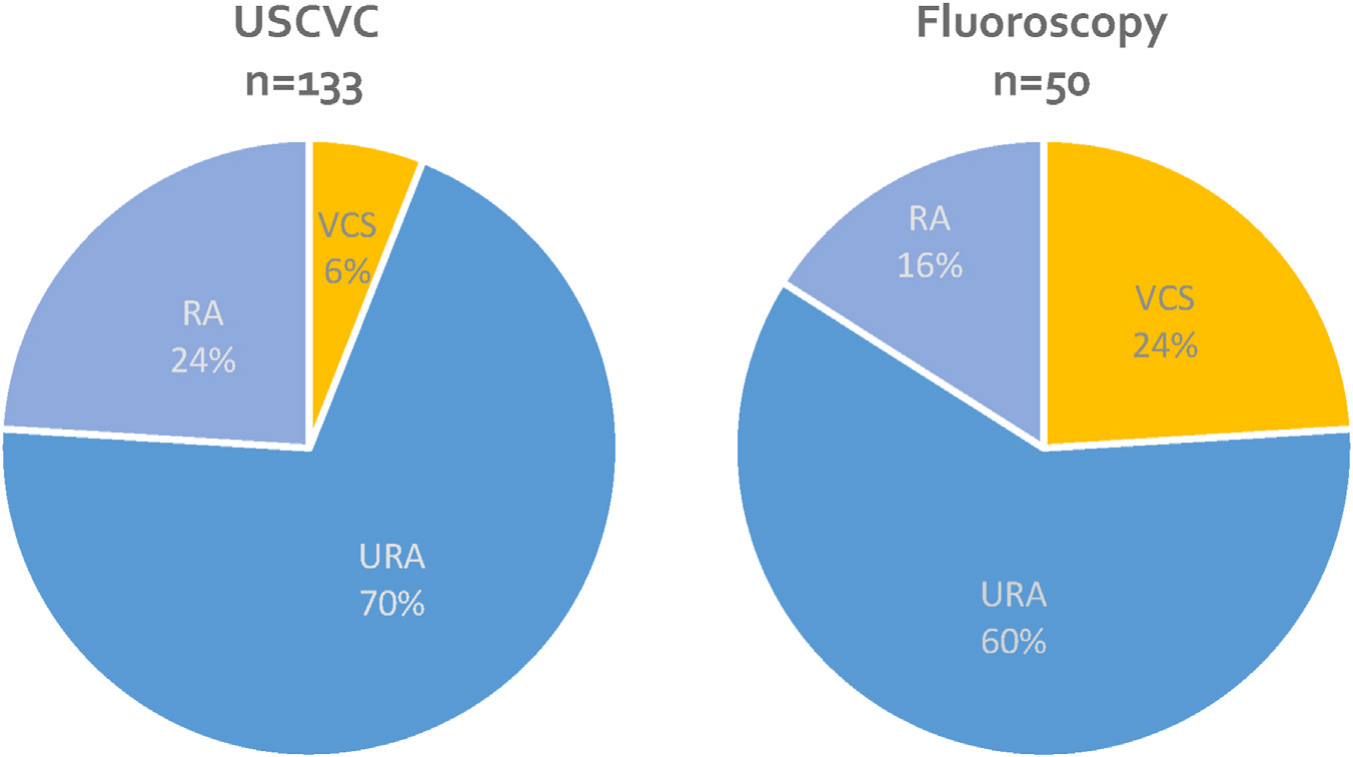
Catheter tip position after ultrasound-guided (*N* = 133) and fluoroscopy-guided catheter placement, *P* = 0.0005, Pearson’s chi-square. RA, right atrium; VCS, vena cava superior; URA, upper right atrium; USCVC, ultrasound-guided central venous catheter.
